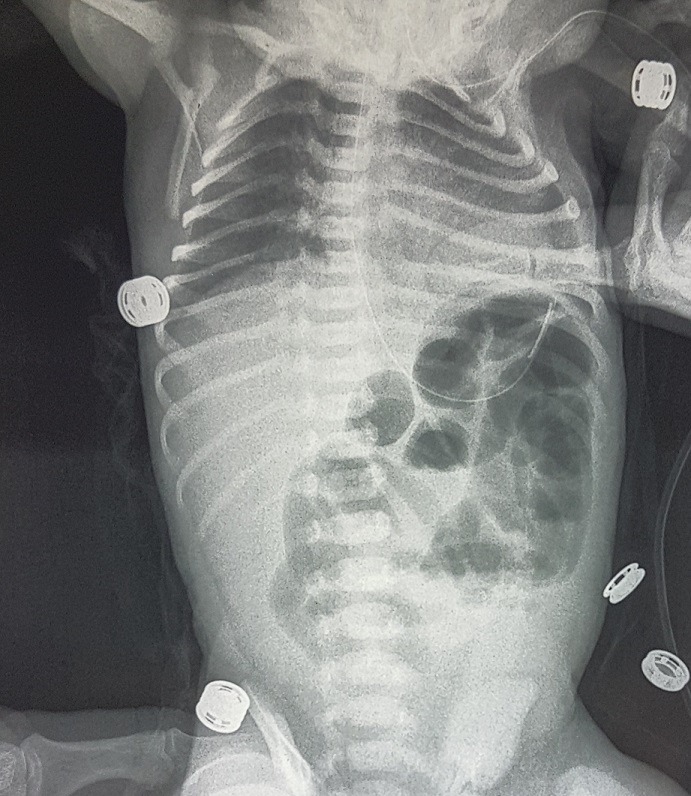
plain abdominal radiograph reveals air fluid levels and dilated loops of small bowel
